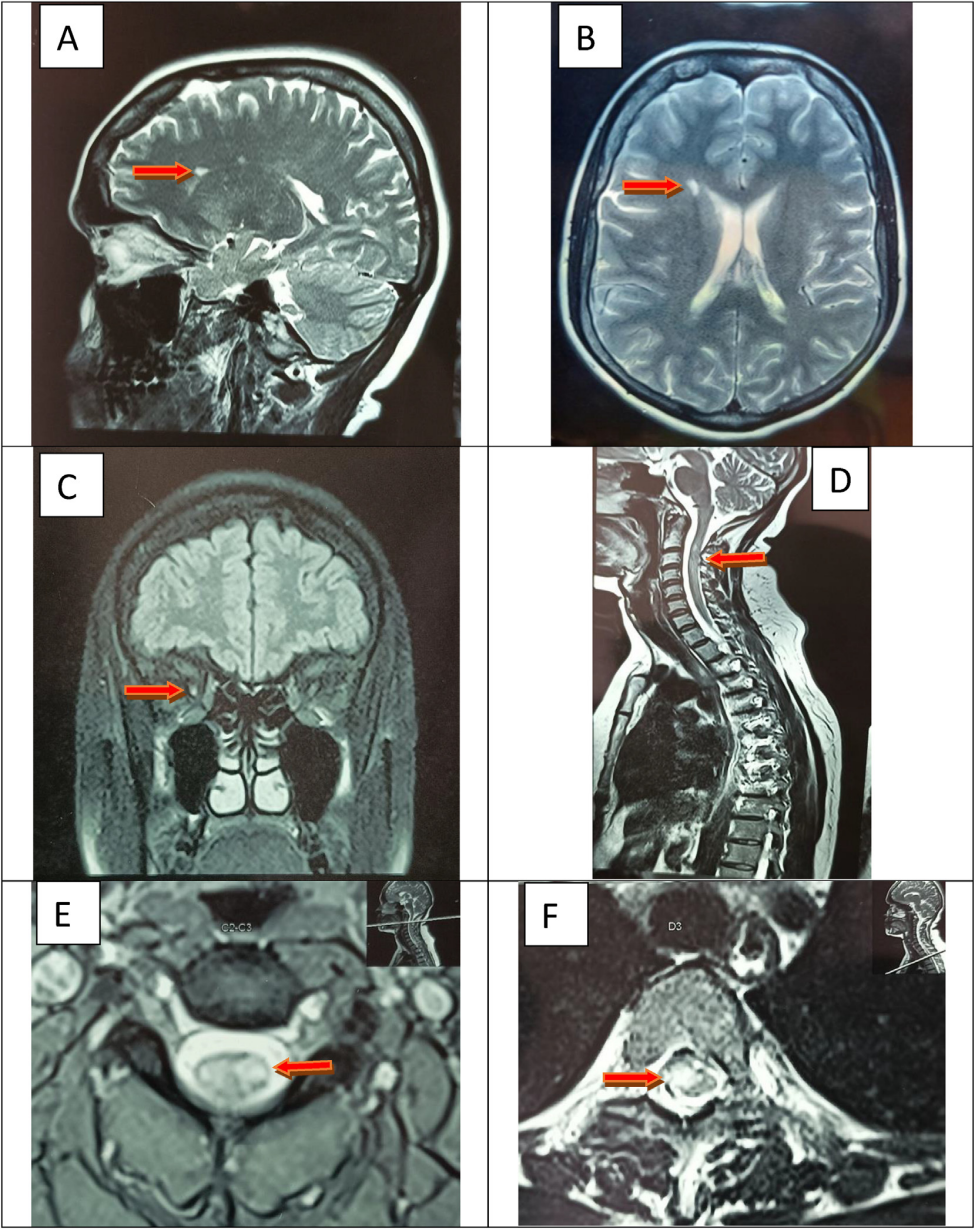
Brain magnetic resonance imaging (MRI) findings. Few hyperintense periventricular lesions were observed in T2 weighted images (A: Sagittal image & B: Axial image). Hyperintense signals in the right optic nerve on T2 weighted image (C: Coronal image). Hyperintense lesions in the C1, C2 & C3 on the left side (D: Sagittal image & E: Axial image) and D3 on the right side on T2 weighted image (F: Axial image).
